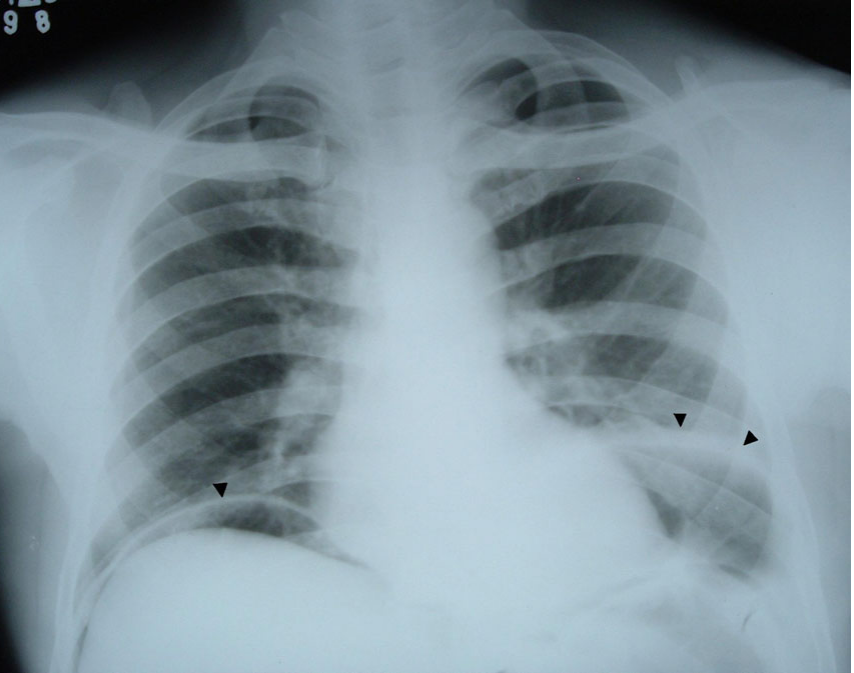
Chest X-ray showing free air under diaphragm (single arrow head) along with the Bochdalek hernia on the right side (double arrow head).
